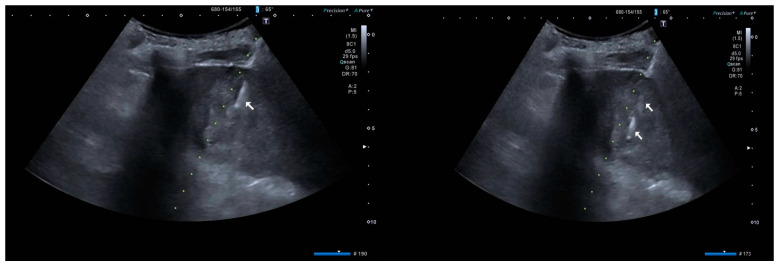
Ultrasound image of biopsied liver segment VI lesion. The arrow indicates the presence of the VI liver segment.
